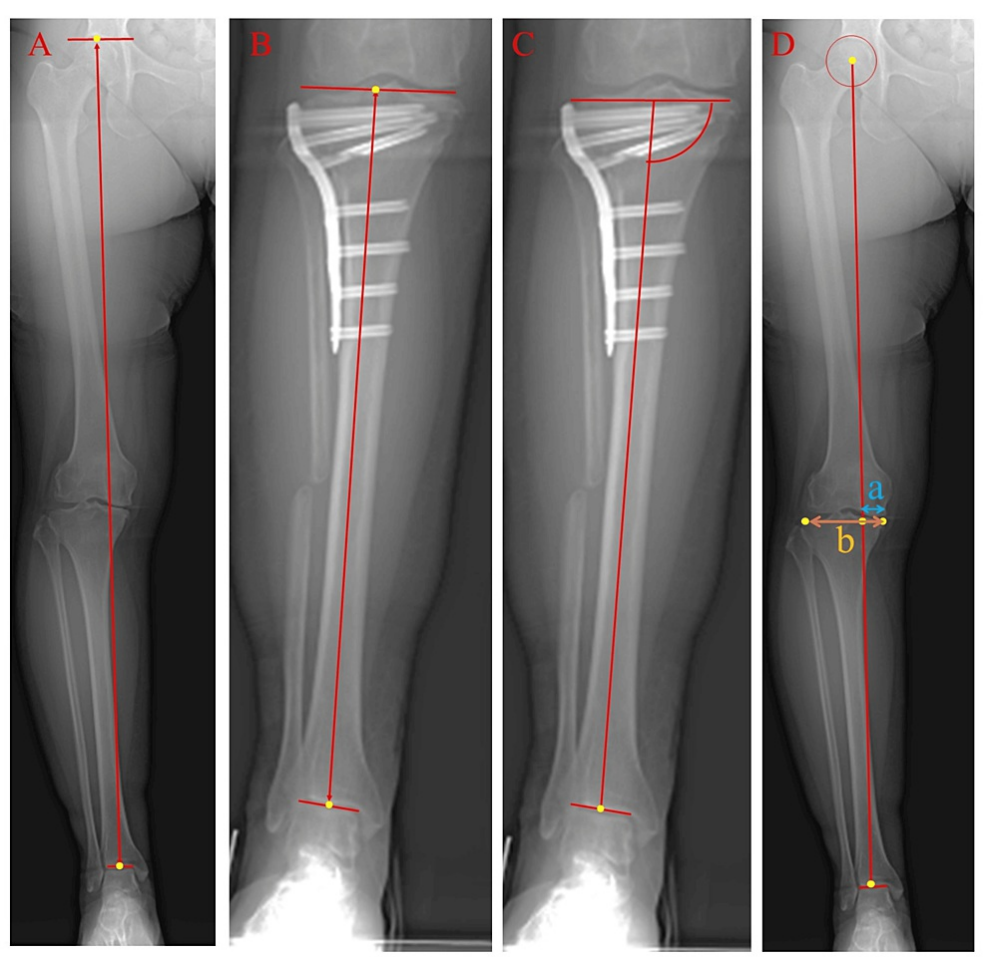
Measurements of lower leg parameters on single-leg-stance anteroposterior radiographs (a) The length of the lower leg was defined as the length of the line from the top of the femoral head to the center of the midpoint of the tibial talar joint; (b) The length of the tibia was defined as the length of the line from the midpoint of the tibial eminences to the center of the midpoint of the tibial talar joint; (c) The medial proximal tibial angle was defined as the angle between the tibial mechanical axis and the articular surface of the proximal tibia; (d) % Mechanical axis was calculated as follows: a/b × 100.
